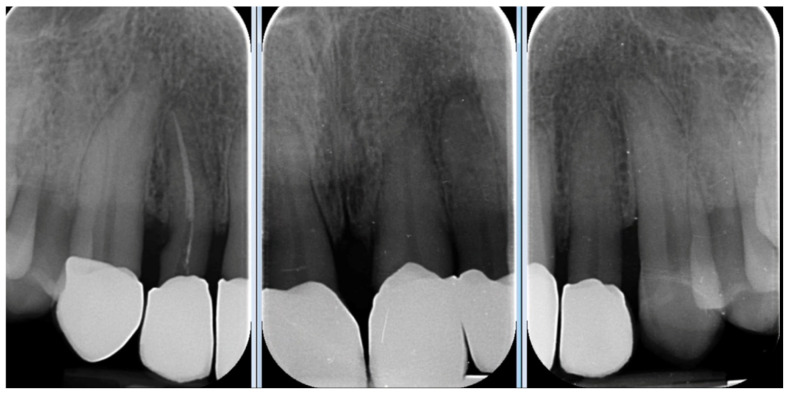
Four-year follow-up radiographic examination.
